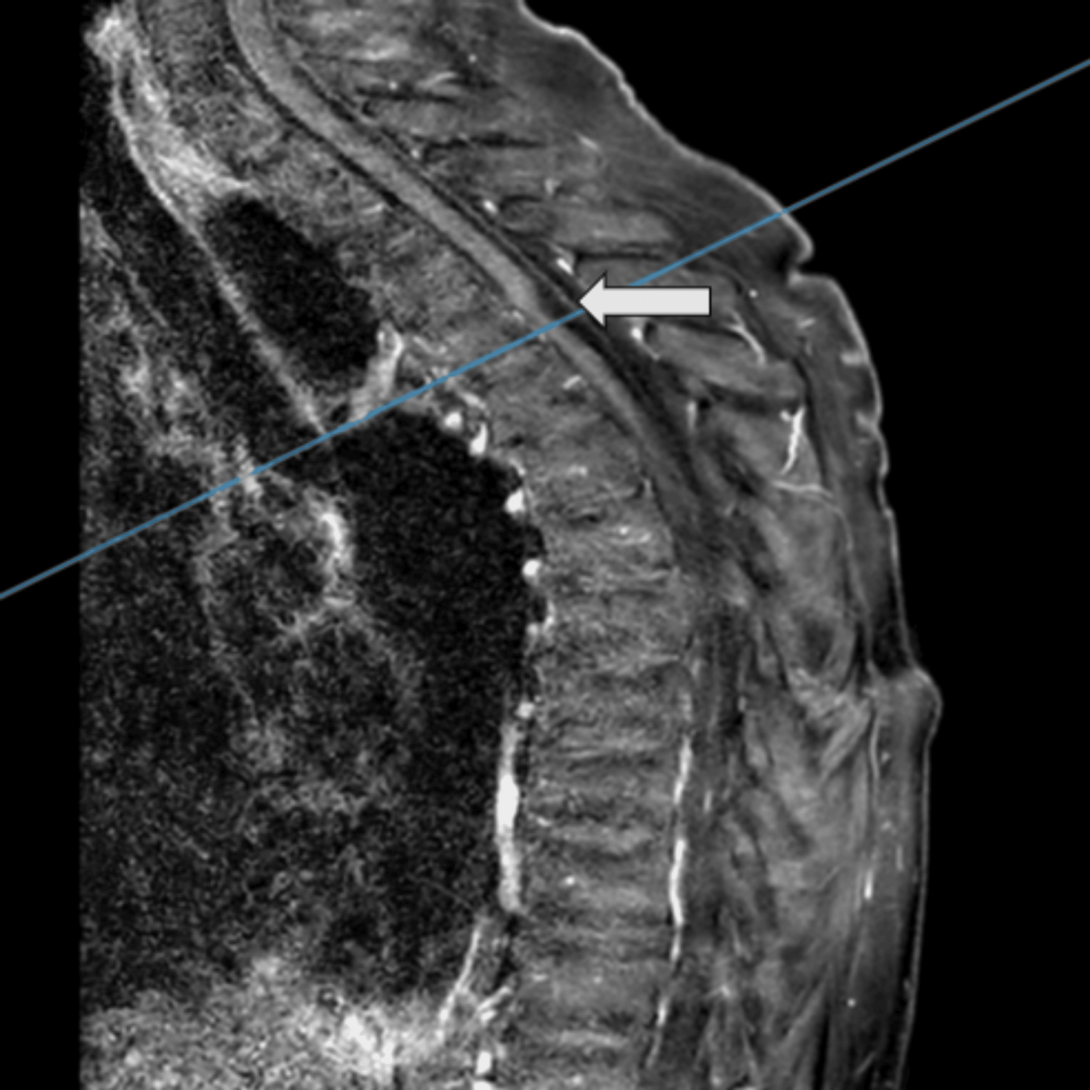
Preoperative T1-weighted MR image with contrast in sagittal view (arrow showing the intraspinal mass, compressing the spinal cord at the T4 level) MR: magnetic resonance
